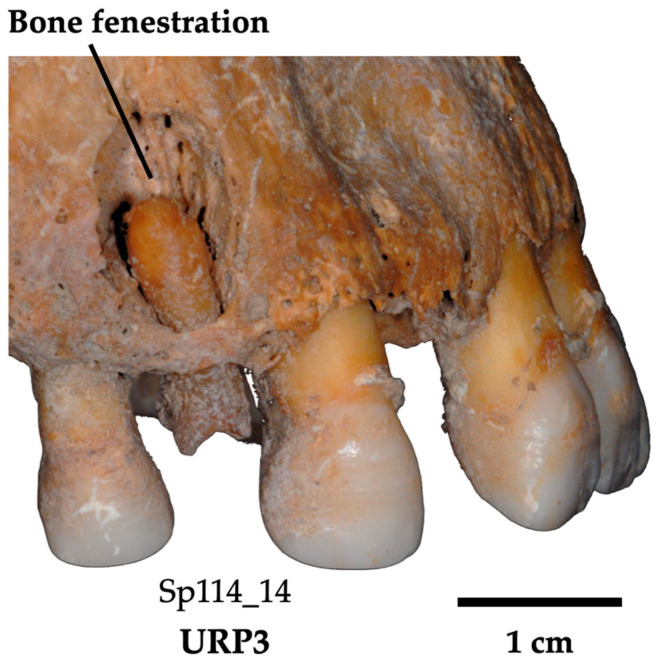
Bone fenestration in the upper left sector of individual Sp114. The destruction of the bone in the periapical area of this URP3 is caused by an infection (i.e., dental periapical abscess) due to a carious lesion with pulp exposure.
